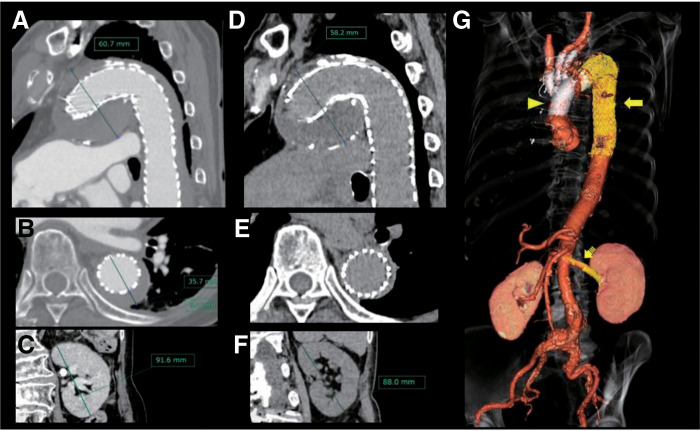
Fig. 3 CT image at 1 week and 3 months postoperatively; 3D reconstruction at 1 week postoperatively. On the postoperative CT image obtained on day 7, the maximum short-axis diameter of the distal arch aneurysm measured 60.7 mm (A), and the false lumen at the Th8 level measured 9.0 mm (B). At 3 months postoperatively, the maximum short-axis diameter of the distal arch aneurysm had slightly reduced to 58.2 mm (D), and the false lumen at the Th8 level had almost disappeared (E). The 3D reconstructed image of the CT on postoperative day 7 shows the triangle representing the prosthetic graft used for TAR+ET, and the arrow indicates the TEVAR stent. The dotted arrow shows the stent in the left renal artery, demonstrating contrast enhancement in the left kidney (G). In the delayed phase of the contrast-enhanced CT performed on postoperative day 7, the left kidney demonstrated contrast enhancement. (C), and no significant changes in kidney size or morphology were observed at 3 months (F). 3D: 3 dimensional; CT: computed tomography; ET: elephant trunk; TAR: total arch replacement; TEVAR: thoracic endovascular aortic repair
